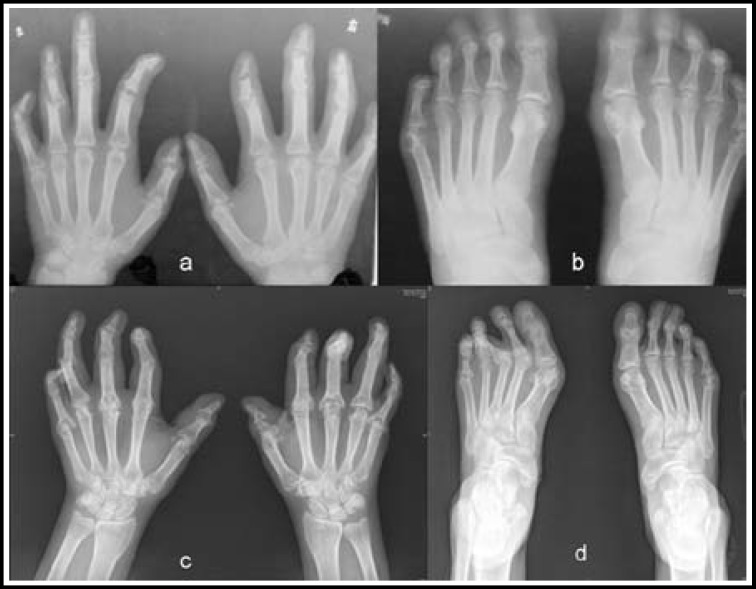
a and b: Radiographs obtained in 1992 showed arthritis in multiple small joints, with bone erosion, in both hands and feet.
